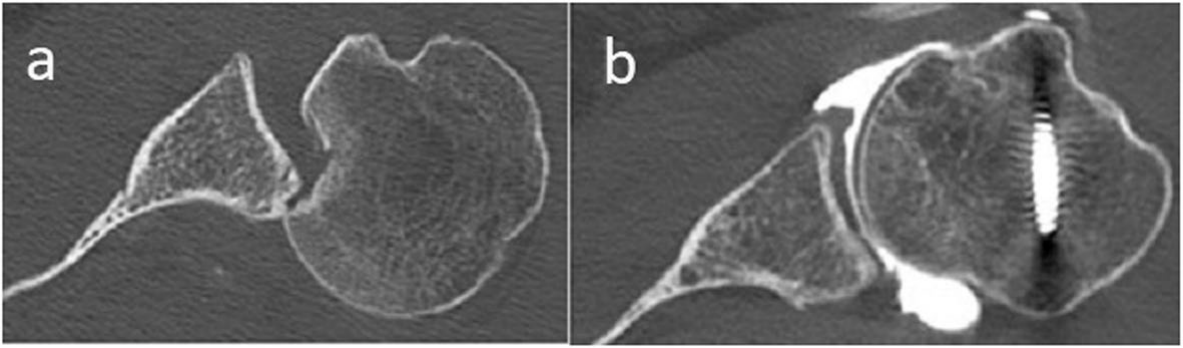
Locked posterior shoulder dislocation (left shoulder), pre- versus postoperative imaging. a Preoperative and (b) postoperative axial computed tomography images of a the left shoulder of a patient with locked posterior shoulder dislocation treated with the modified McLaughlin procedure
